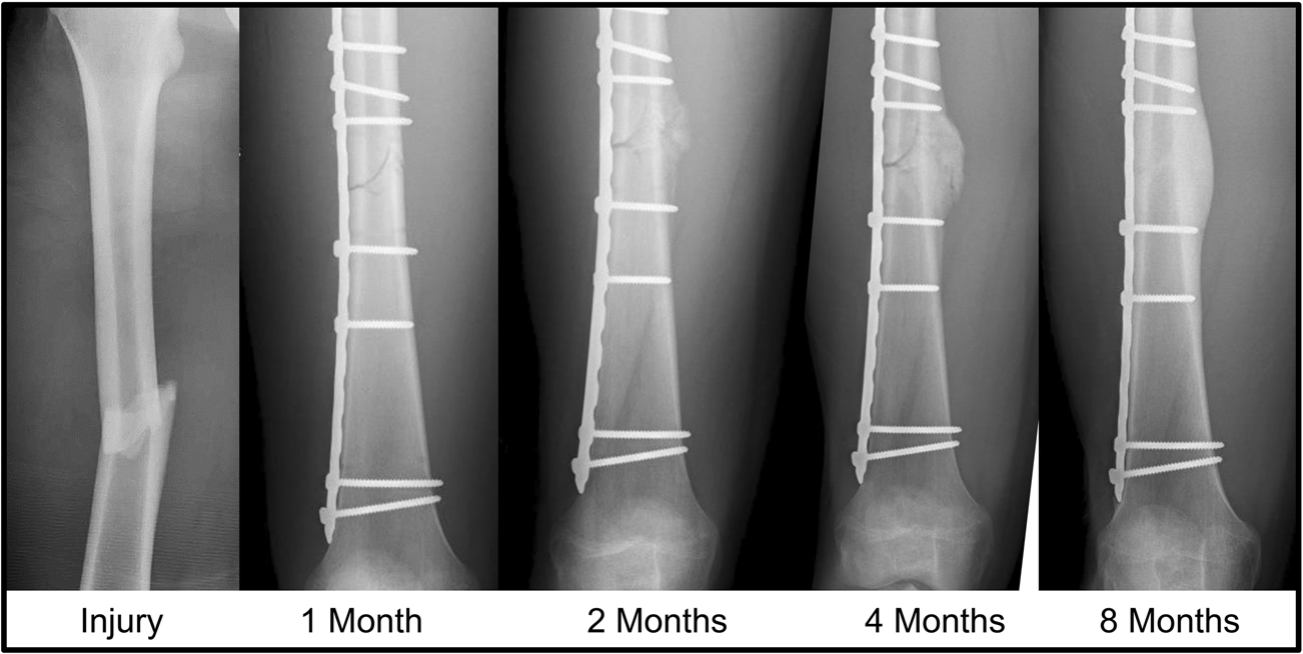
Radiographs as “angiograms.” These radiographs depict a healing femur fracture in a young adult treated with open reduction and internal fixation with plate and screws. The hardware provides greater stabilization on the ipsilateral side of the fracture and more chondroid soft tissue callus is required on the contralateral fracture side for equivalent stabilization. The lack of motion on the side of the plate coupled with the compression of the fracture prevents callus formation. The hazy soft tissue callus become radiopaque as it is replaced by hard tissue, which is definitive evidence of vascular ingress to the area
